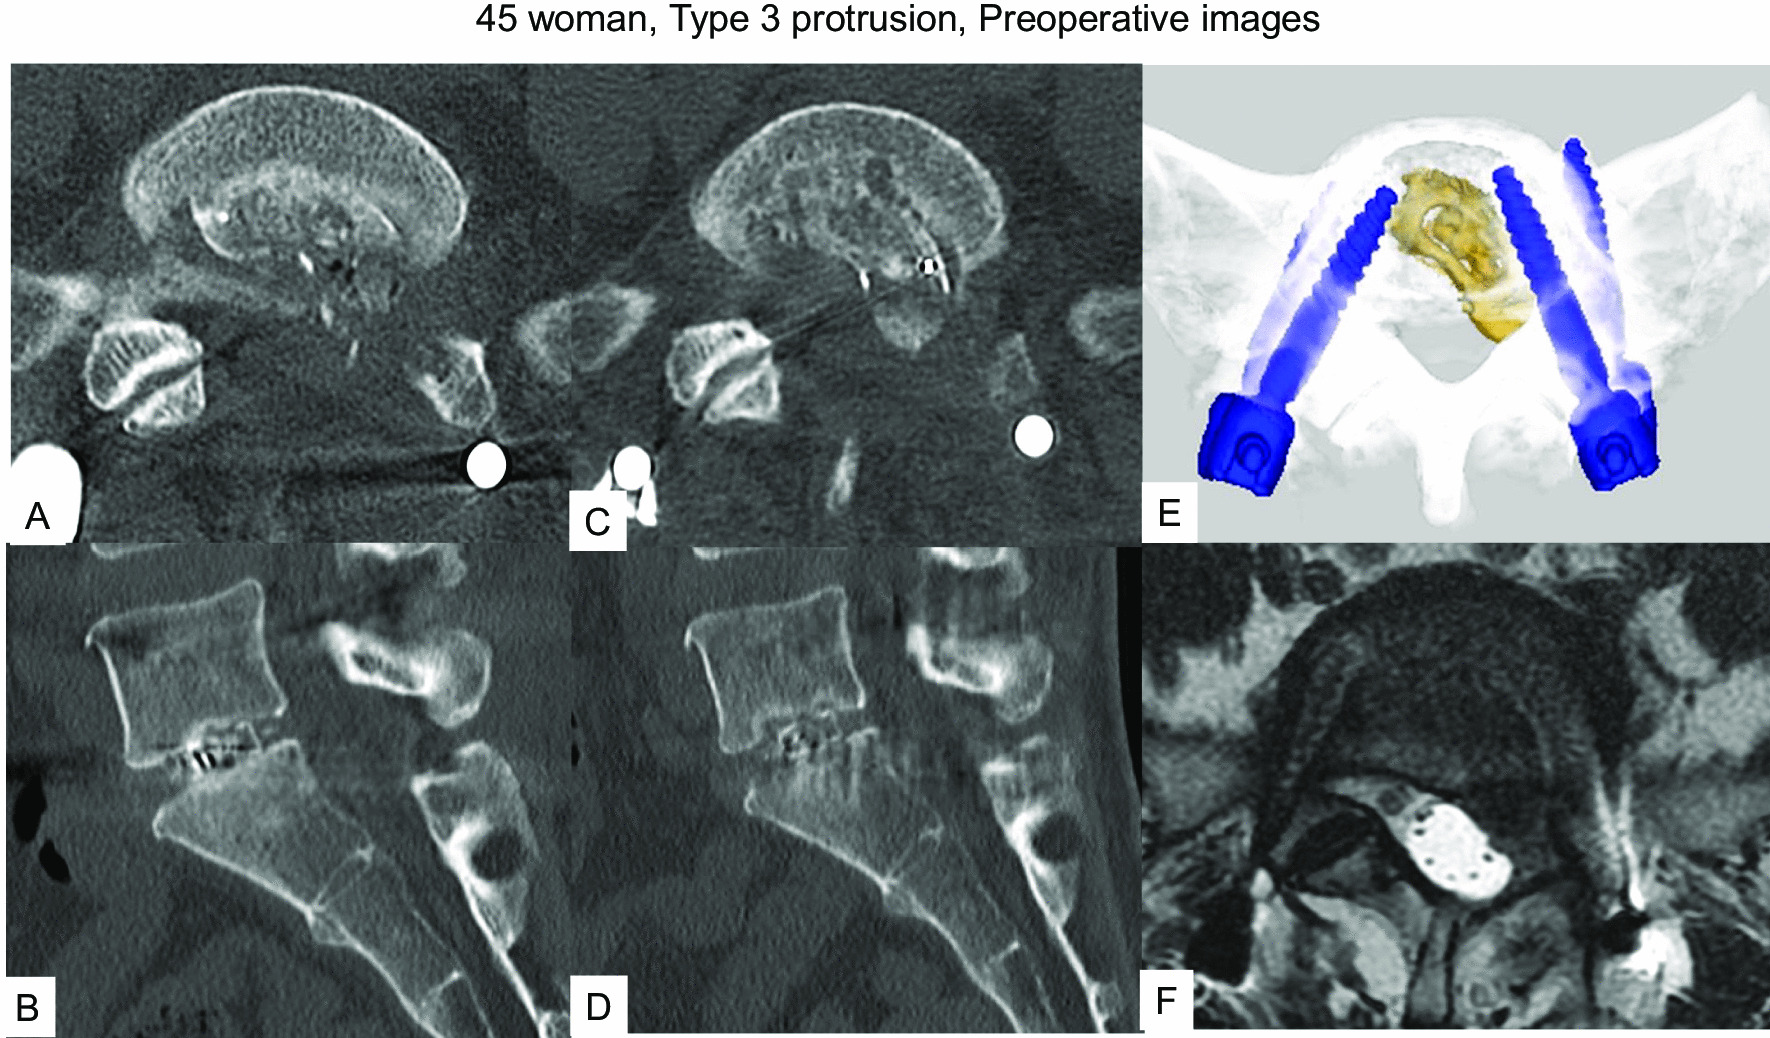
45 woman, Type 3 protrusion, images before revision surgery A, B Images at one month follow-up, A Axial CT at L5/S1, B Sagittal reconstruction CT, C–F Images at three months follow-up, C Axial CT at L5/S1, D Sagittal reconstruction CT, E 3-D CT, F Axial T2-weighted MR imaging at L5/S1
